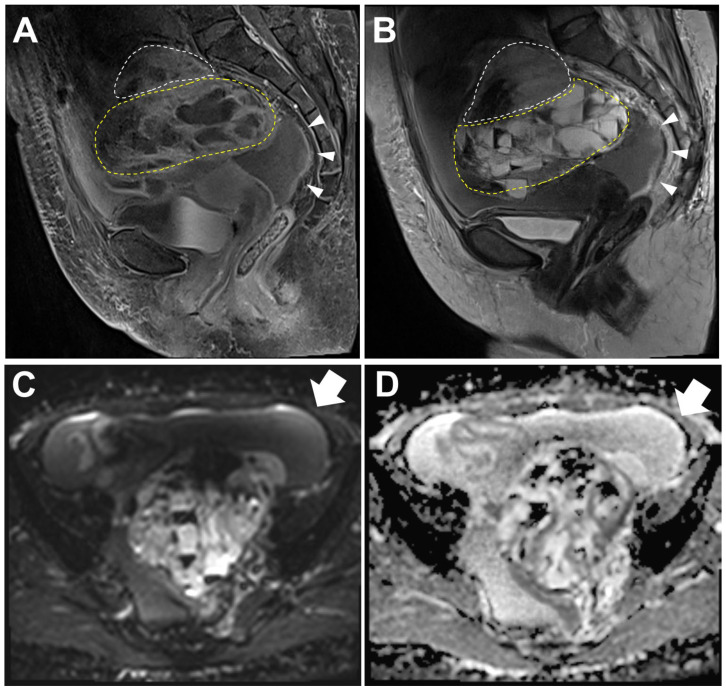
Magnetic resonance imaging (MRI) of the pelvis. Sagittal fat suppression T1-weighted (A) and T2-weighted (B) images revealed a multiloculated cyst with watery fluid or internal hemorrhage in the left ovary measuring 11.7 cm in size (anterior–posterior extension: 11.1 cm, width: 10.5 cm, oblique craniocaudal extension: 11.7 cm). Based on the multilocular cystic appearance with a macrofollicular pattern filled with watery fluid or hemorrhage, the impression was an adult granulosa cell tumor (AGCT) [1,2,3] and endometrioid carcinoma [4]. A solid component was noted at the cranial side (white dashed line) of the mass, measuring 5.4 cm in size (anterior–posterior extension: 4.0 cm, width: 5.4 cm, oblique craniocaudal extension: 3.5 cm). In this area, fat suppression T1-weighted images exhibited enhancement resembling that of a multilocular cyst (yellow dashed line), whereas the T2-weighted images showed a comparatively lower level of enhancement. In addition, peritoneal thickening was shown (arrowheads). Diffusion-weighted image (C) and apparent diffusion coefficient map (D) showed ascitic fluid without diffusion restriction (arrow).
